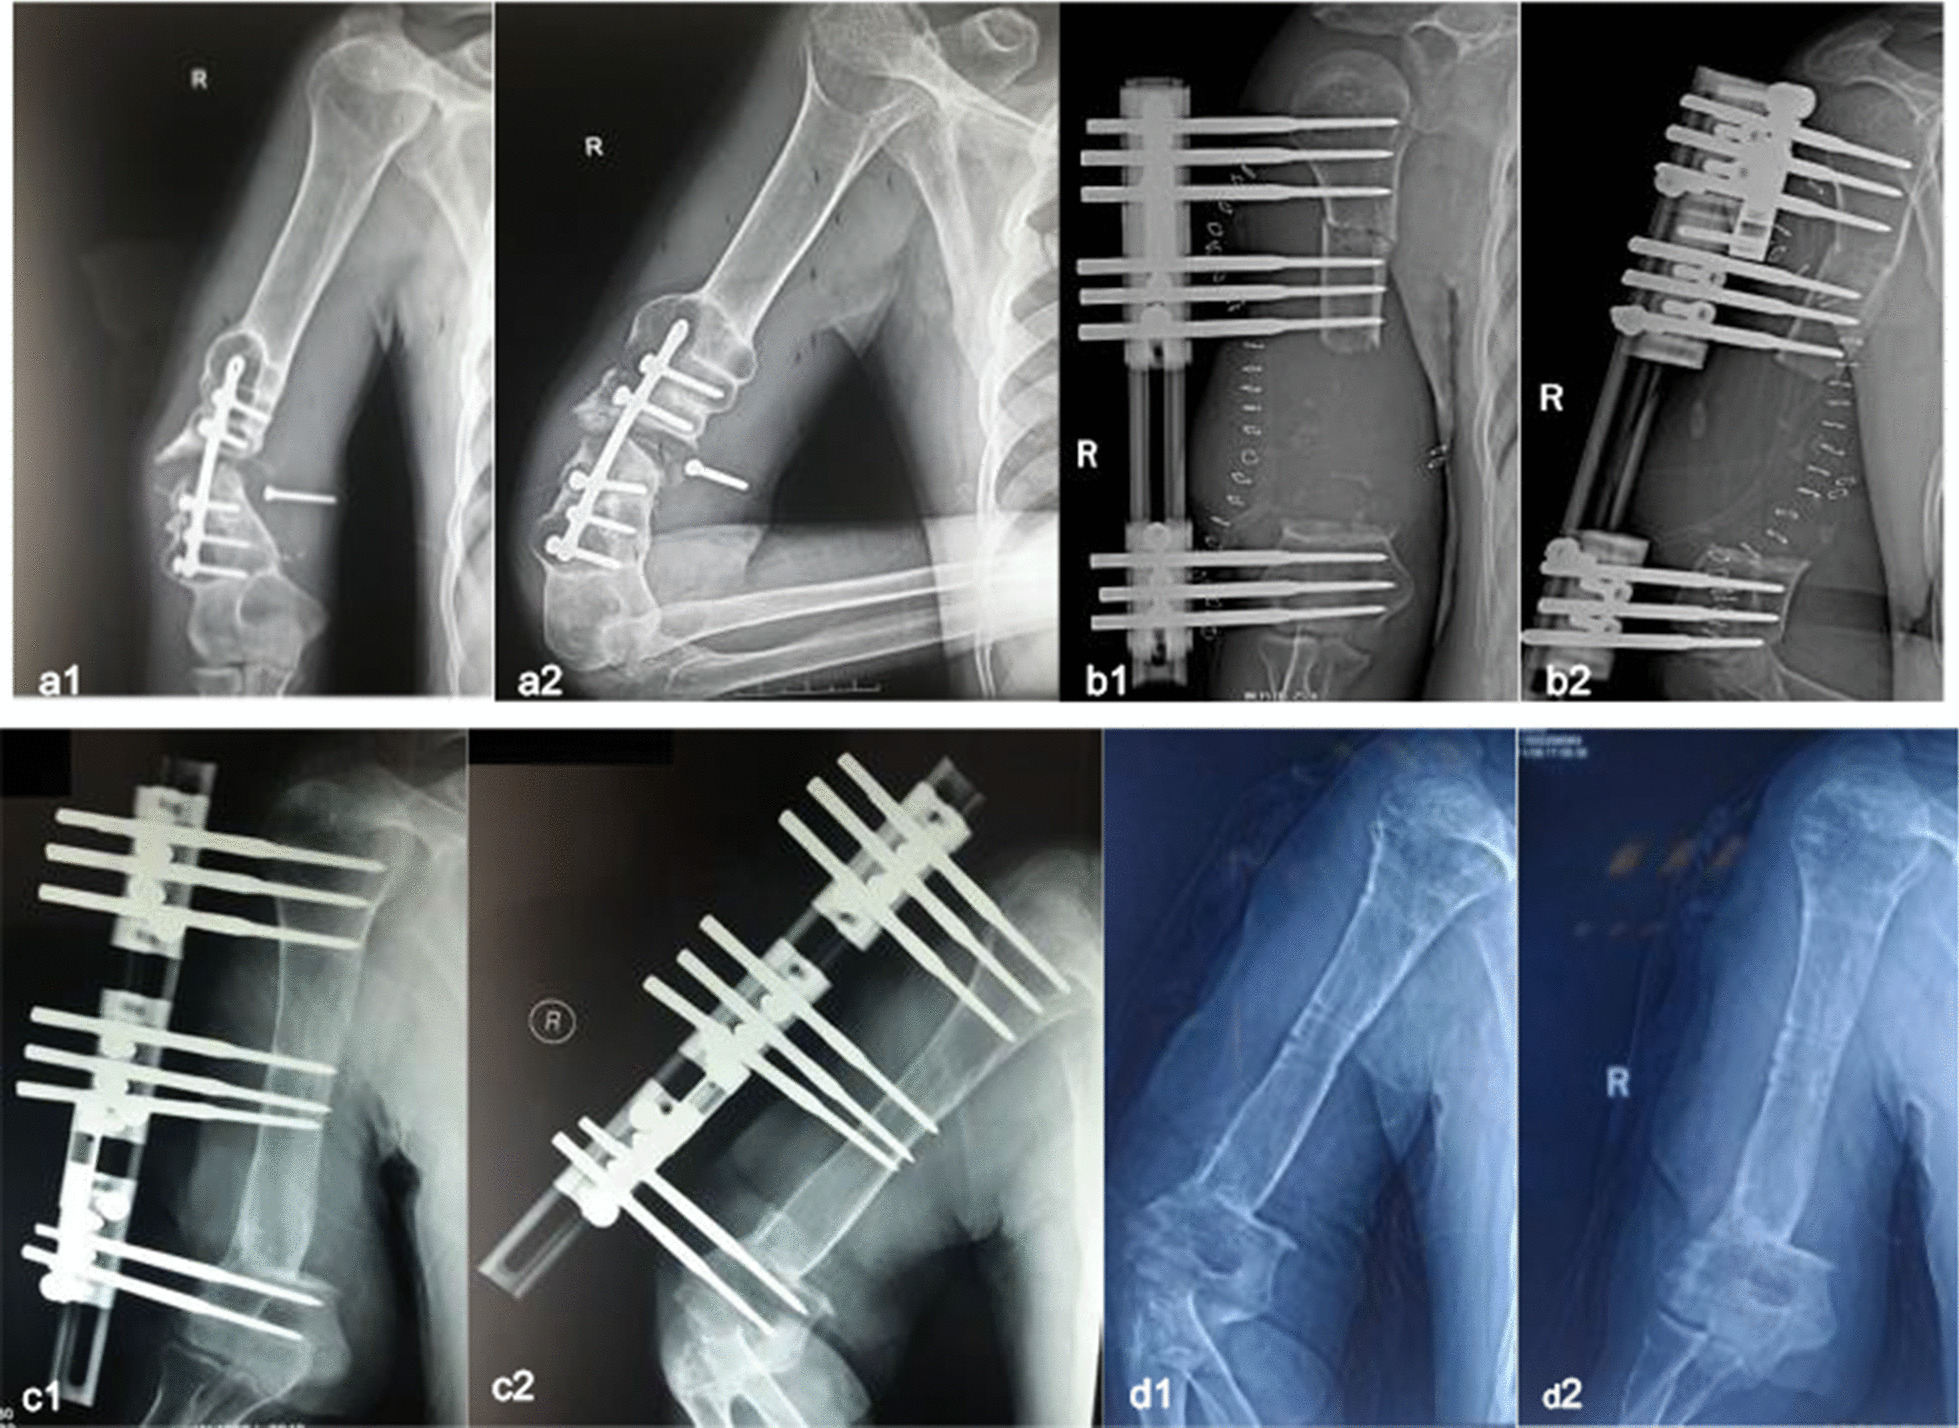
Radiographs of this patient at different stages of distraction osteogenesis treatment. a1, a2 Preoperative. b1, b2 Postoperative. c1, c2 8 months postoperatively. d1, d2 12 months after removal of the external fixator
